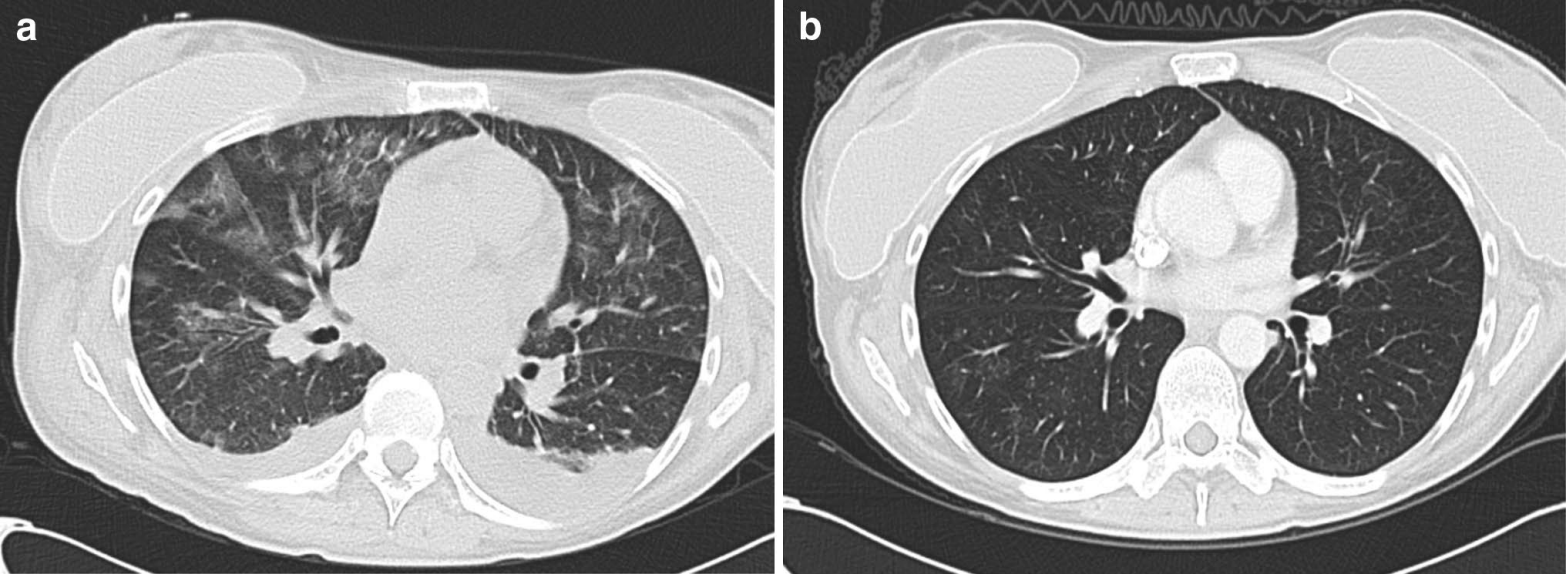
Evidence of pulmonary infiltrates consistent with local cytokine release syndrome in patient 36,013. CT images demonstrating non-specific pulmonary infiltrates 10 days after MFEζ T cell infusion (a) and resolution by day 42 (b)
